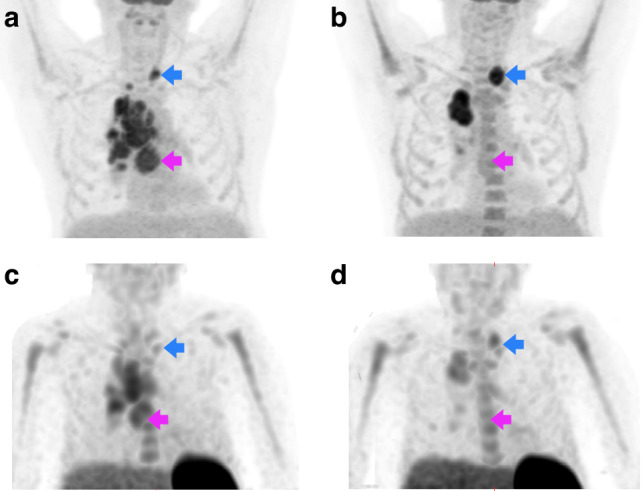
Changes in [^99m^Tc]NM-01 uptake may be associated with metabolic response. Maximum intensity projection images of (a) baseline and (b) 9-weeks [^18^F]FDG PET/CT and (c) baseline and (d) 9-weeks [^99m^Tc]NM-01 SPECT/CT in a 64 year old male with PD-L1 positive (PD-L1 TPS 80%; from stations 4 R, 4 L and 7 thoracic lymph nodes) metastatic NSCLC and dissociated metabolic response at 9-weeks following single agent anti-PD-1 pembrolizumab. For example, a subcarinal (station 7) node metastasis responds to treatment, with a baseline (a) [^18^F]FDG SUV_max_ of 9.1 and (c) [^99m^Tc]NM-01 T:BP of 4.81, and 9-weeks (b) SUV_max_ 3.4 and (d) [^99m^Tc]NM-01 T:BP of 1.41 (pink arrows). However, a left supraclavicular fossa node does not respond to treatment, with a baseline (a) [^18^F]FDG SUV_max_ of 8.5 and (c) [^99m^Tc]NM-01 T:BP of 2.08, and 9-weeks (b) SUV_max_ 12.2 and (d) [^99m^Tc]NM-01 T:BP of 2.74 (blue arrows).
